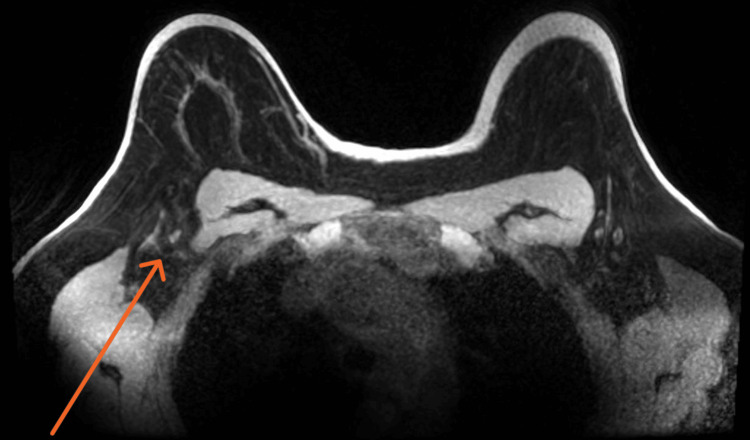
Post-chemotheraphy MRI of right axillary adenopathy (orange arrow) does not show cortical thickening.
